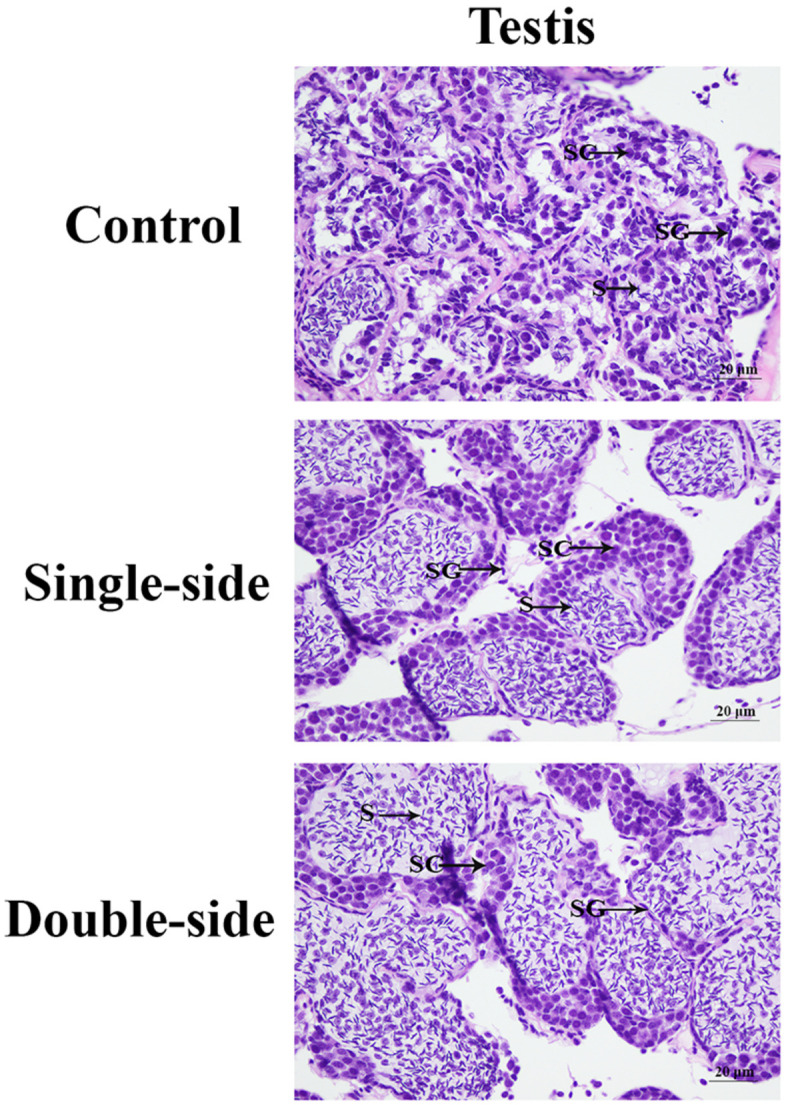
The morphological differences of the testis after the ablation of eyestalk. SG, spermatogonia; SC, spermatocytes; S, sperms; and CT, collected tissue. Scale bars = 20 μm.
